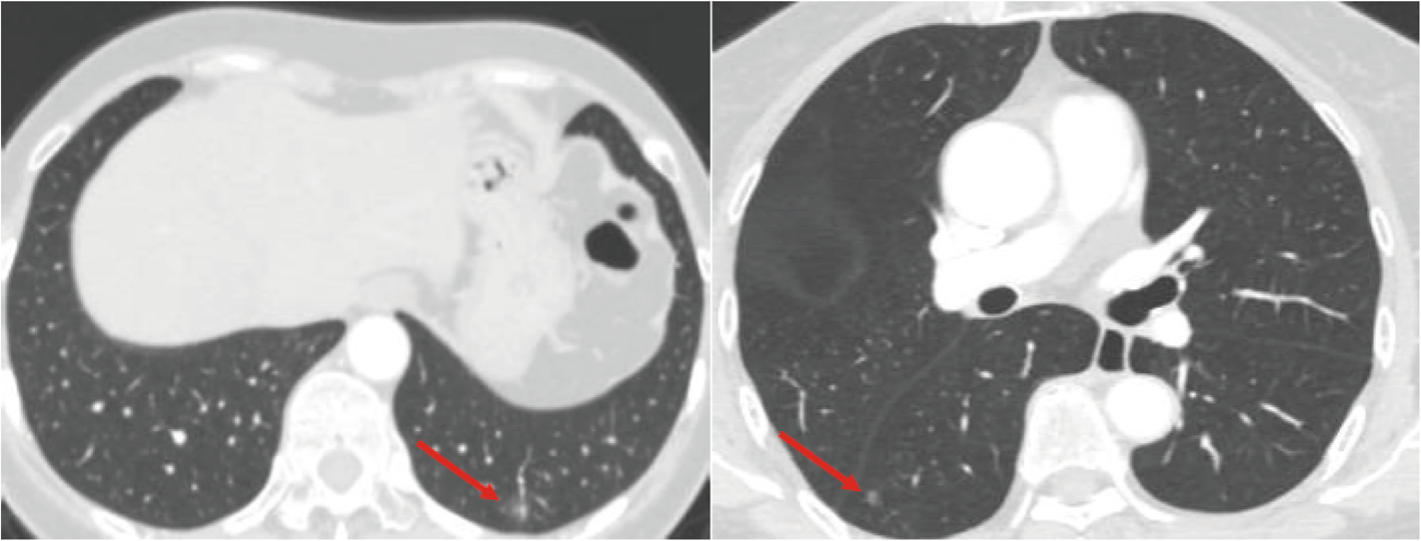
CT images of 2 CMPT patients treated at our hospital (left side: case 1, right side: case 2)
